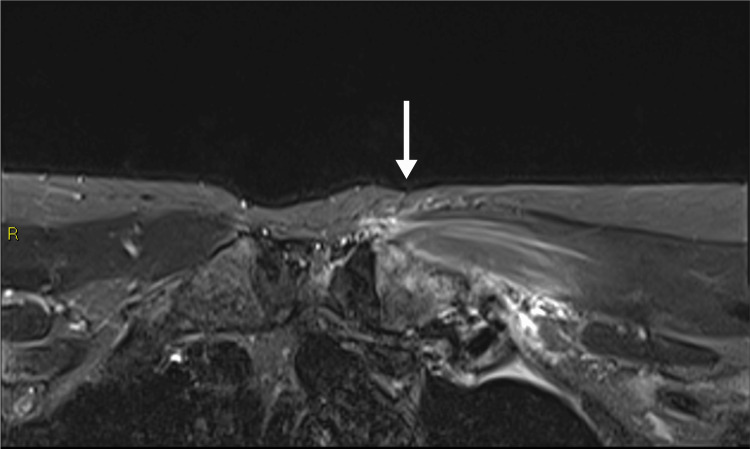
Axial fat suppressed fluid-sensitive MRI shows edema in the left distal first rib and surrounding soft tissue, in keeping with costochondritis. There is also mild signal change in the adjacent medical clavicle head. There is neither significant effusion of the left sternoclavicular joint, nor is there marrow edema in the sternum. The overlying pectoralis major muscle is edematous, likely secondary to reactive change.
